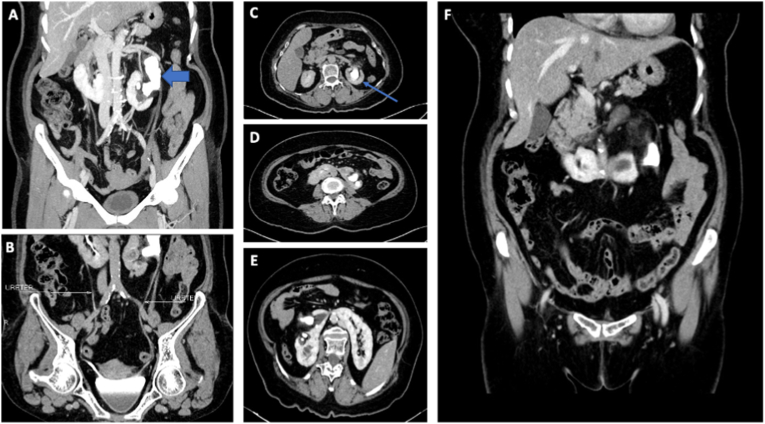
(A) Preoperative CT-Scan showing staghorn stone in left kidney, (B) Preoperative CT-Scan showing normal ureter, (C) Puncture site (superior pole) (D–F) Preoperative CT-Scan showing horseshoe Kidney.
